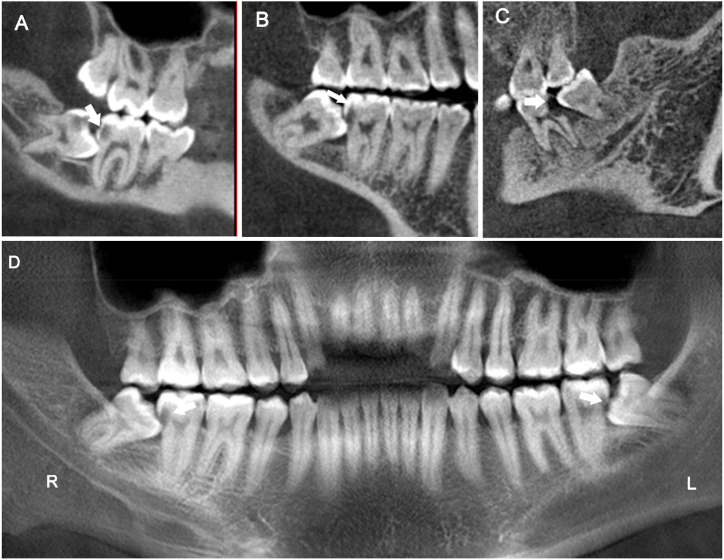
Classification of the severity of distal caries in mandibular second molars (CBCT images, arrows indicate distal caries in MSMs associated with IMTMs). (A) Shallow caries (sagittal section). (B) Moderate caries (sagittal section). (C) Deep caries (sagittal section). (D) The CBCT-generated panoramic image displays shallow caries in the left MSM and moderate caries in the right MSM.
